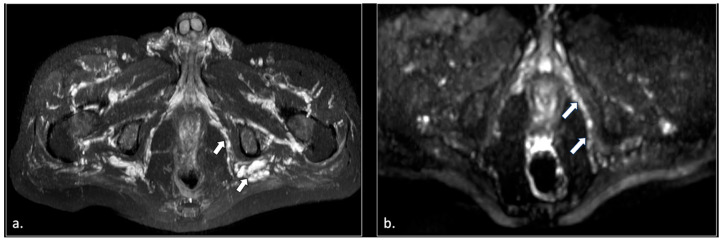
A 71-year-old male with chronic pudendalgia. (a) STIR maximum intensity projection (MIP) scan shows diffuse venous dilatation of the lower pelvis, including varices of pudendal veins (arrow). (b) b600 DWI maximum intensity projection (MIP) shows enlargement and hyperintensity of the left pudendal nerve (arrow).
